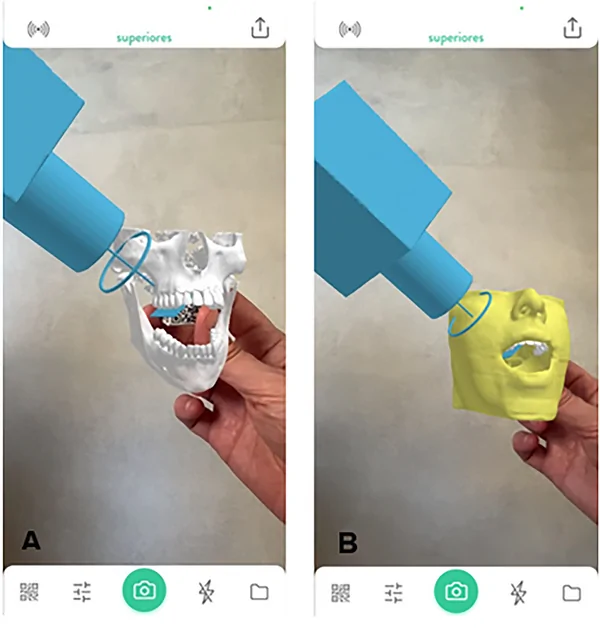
Screenshot of the AR visualization of a radiographic technique, with area and point of incidence.
